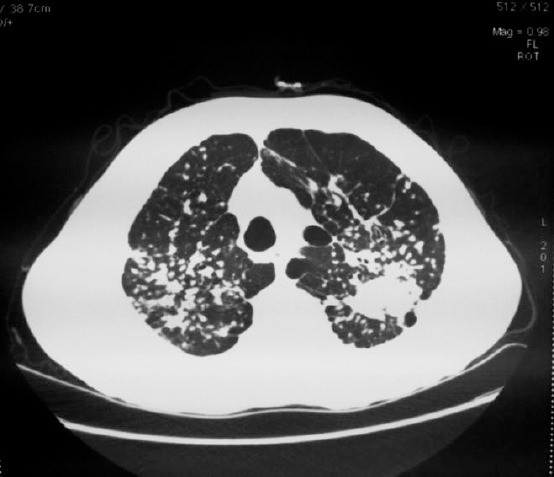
Multislice computed tomography of the thorax*.
